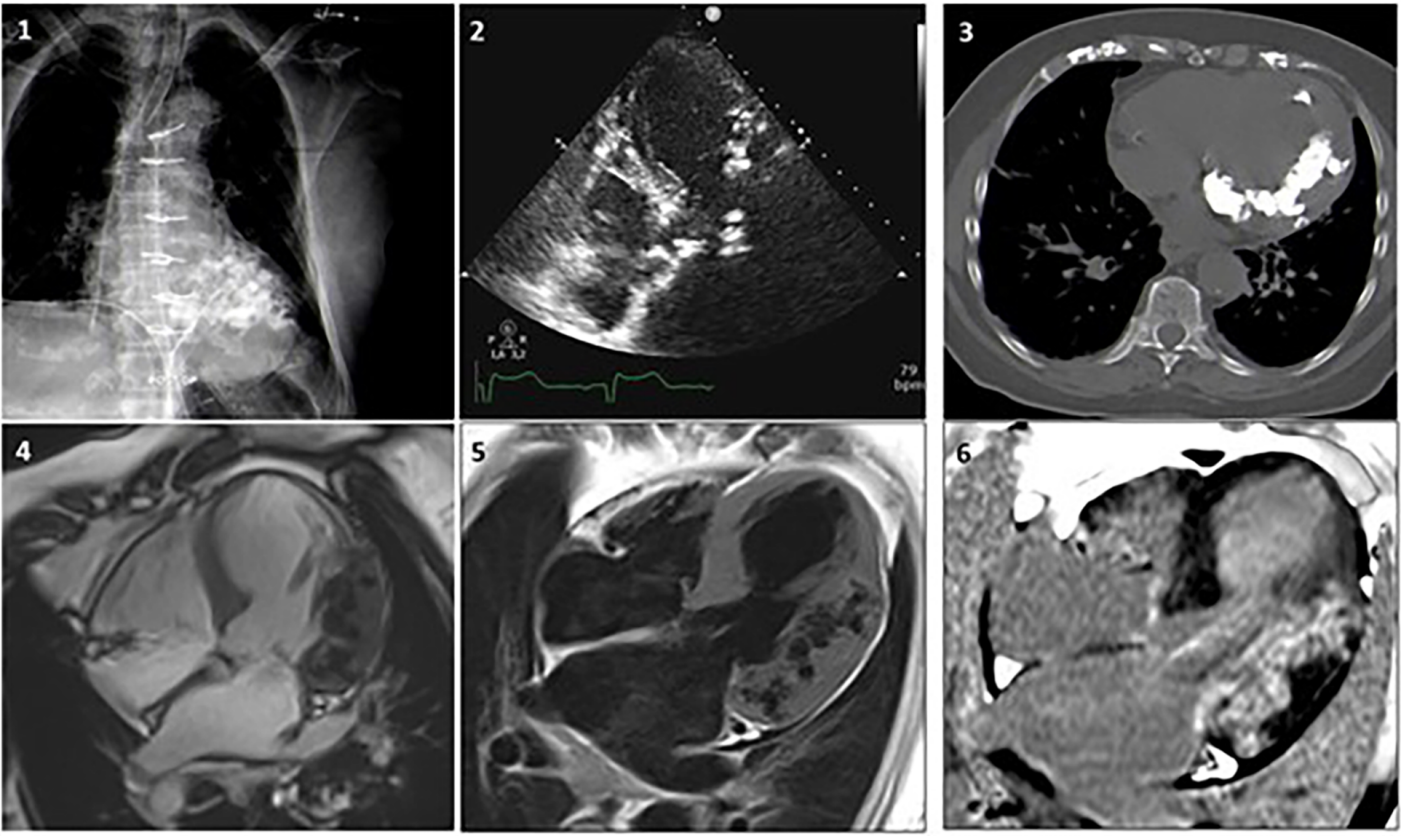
Case 1. A woman, 80 year-old, with CAD underwent revascularization through coronary artery bypass grafiting. (1) Chest x-ray showing a diffuse hypodense lobulated area in the left ventricle. (2) Echocardiogram revealing diffuse aortic and mitral calcifications with mild stenosis, marked septum and antero-lateral asymmetric hypertrophy, with extensive antero-lateral calcifications. (3) Non-contrast CT MPR axial-view presents widespread calcifications in the left ventricular wall extended to the mitral-aortic annulus and both coronary arteries. (4) CMR SSFP 4-chamber view showing lateral and antero-lateral wall thickening with areas of intra-myocardial low signal, surrounded by normal myocardium. Coronary angiography documented significant disease that required revascularization. (5) FSE T1 weighted-spin-echo4-chamber view is confirming the localization of lateral and anterolateral wall thickening with areas of low-signal. (6) After contrast gadolinium injection an extensive area of intra-myocardial hypersignal (hyperenhancement) is shown in the lateral wall. Adapted with permission from Sozzi et al. (21), licensed under CC BY 4.0.
